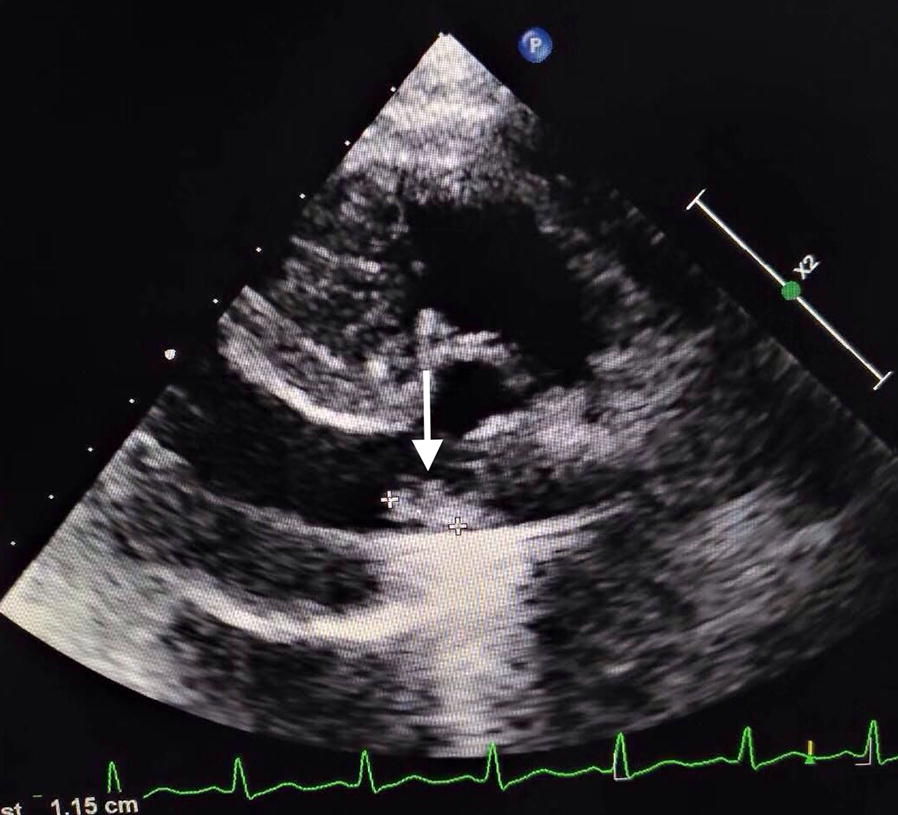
Transoesophageal echocardiogram showing a paravalvular abscess on the prosthetic aortic valve (arrow)
